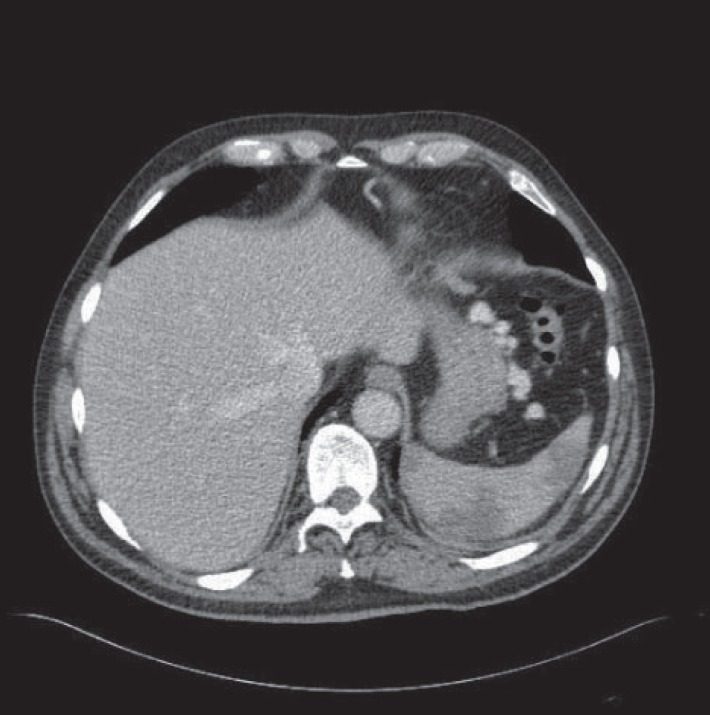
Computed Tomography (portal venous phase images) showing small segmental splenic infarcts (10-20% volume).
